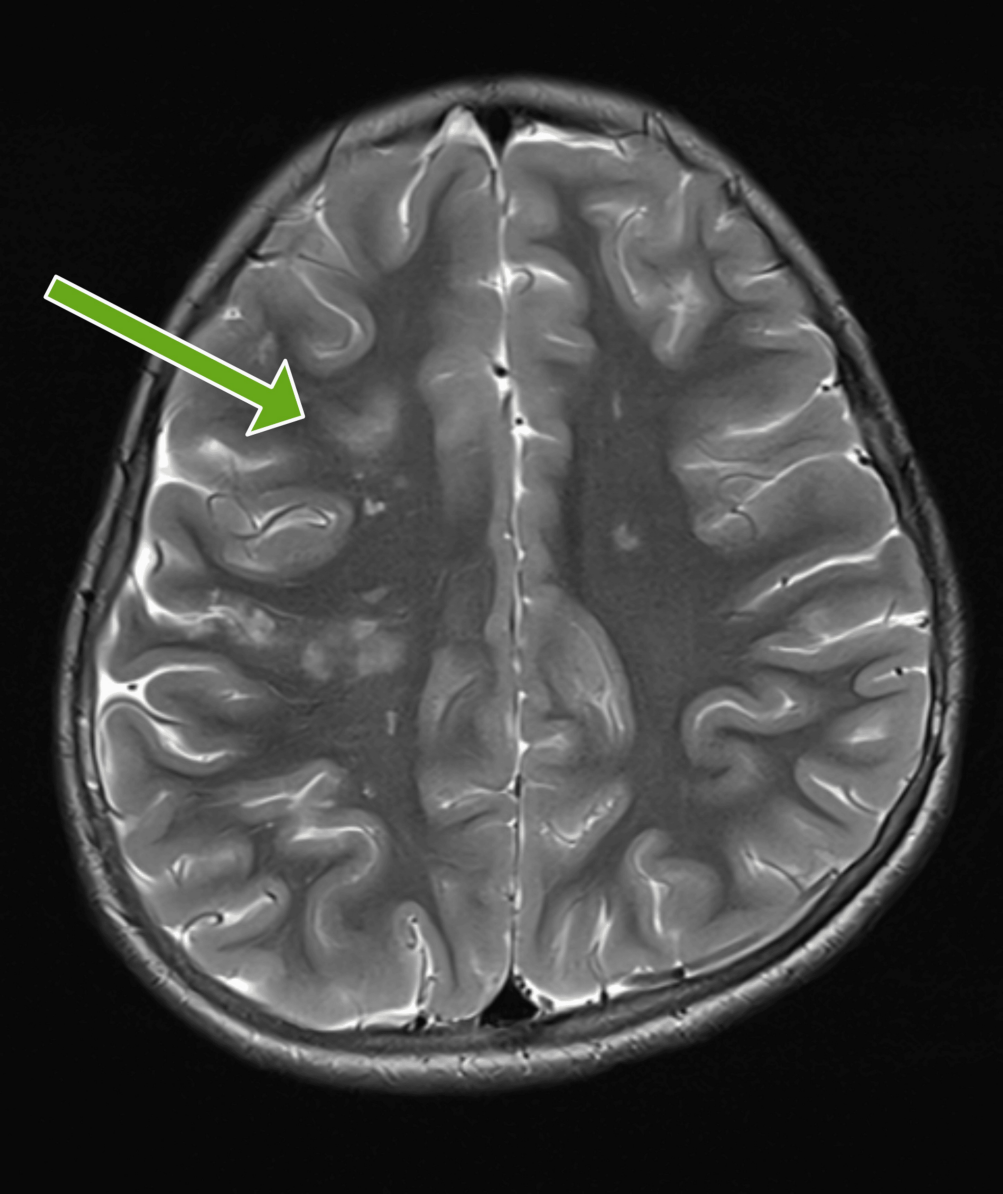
T2-weighted imaging showing hyperintensities in the right frontoparietal region.
